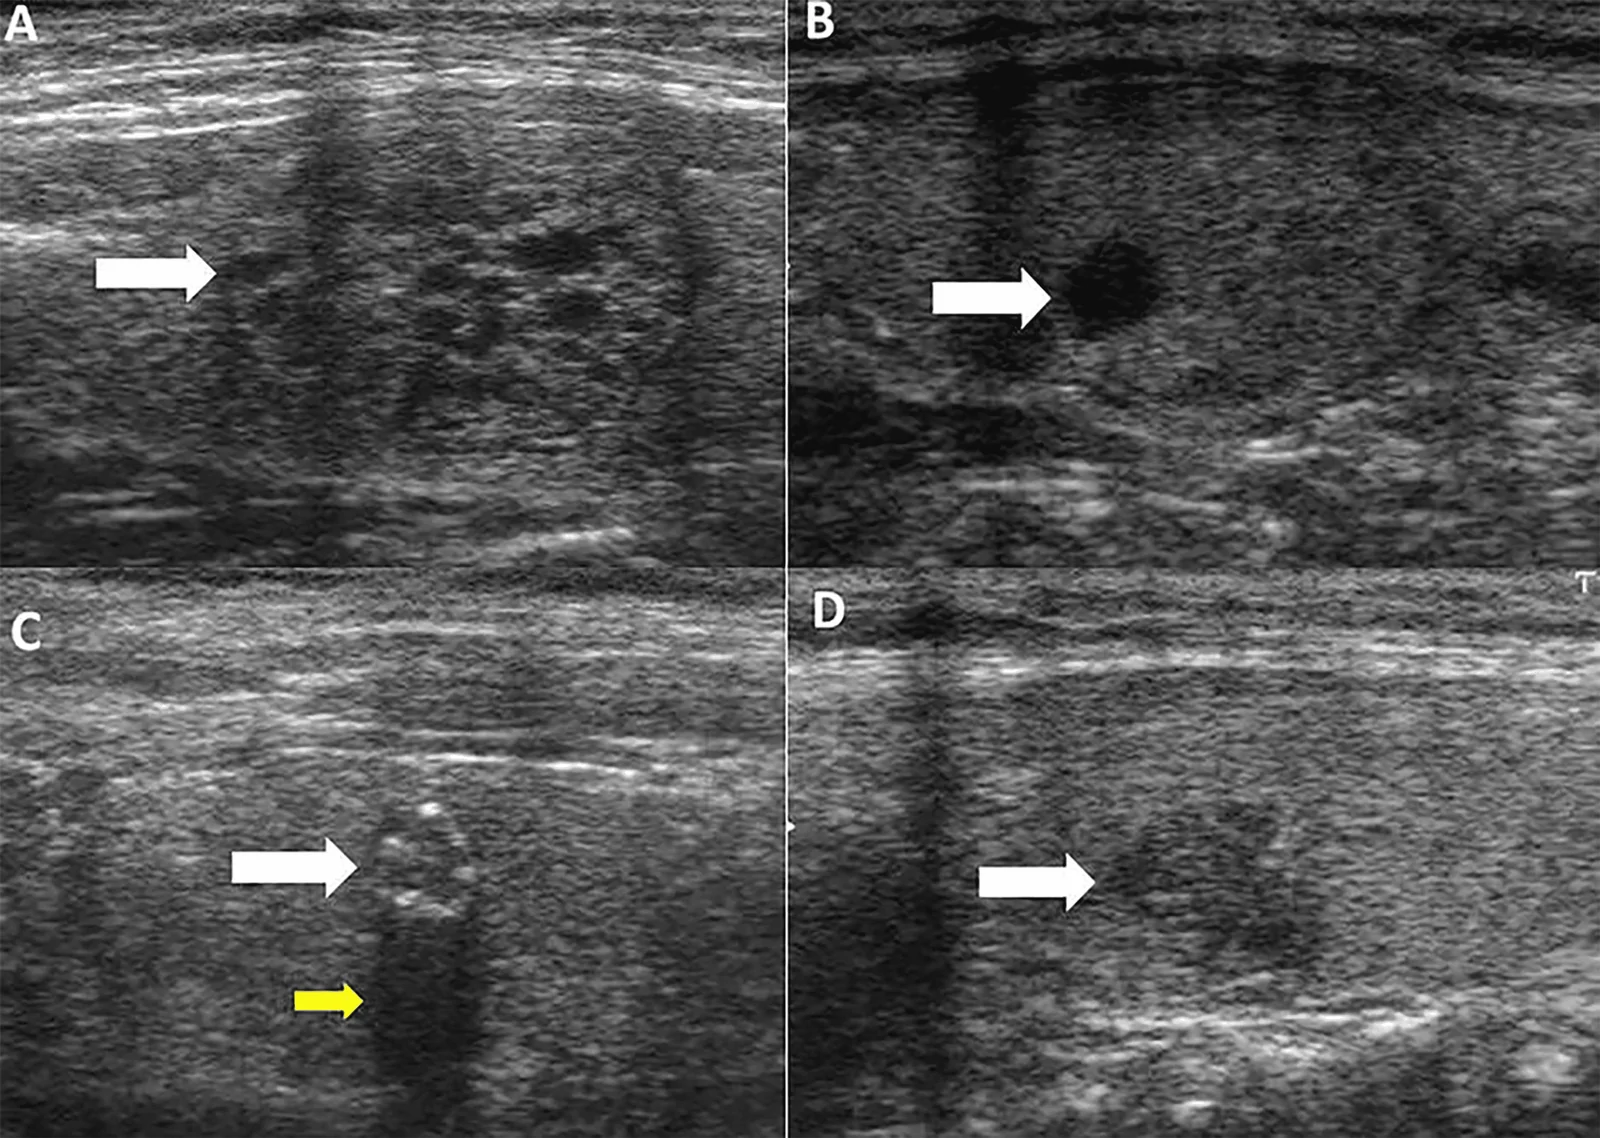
Sonographic features of benign and suspicious thyroid nodules. (A-B) These images depict likely benign nodules, including a well-defined isoechoic nodule (white arrow, A) and a well-defined anechoic cystic nodule (white arrow, B). (C-D) These images depict nodules with suspicious features, including a well-defined hypoechoic solid nodule with calcifications (white arrow, C) and posterior acoustic shadowing (yellow arrow, C), as well as an irregular hypoechoic solid nodule (white arrow, D). Credit: Adapted from Sultan [14], Cureus. Used under CC BY 4.0.
